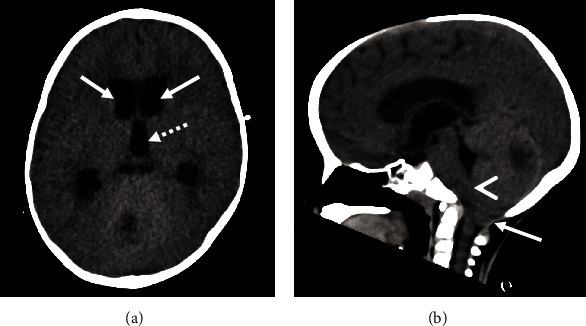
Noncontrast head CT images from hospital day 3 associated with neurological decline. (a) Axial CT image shows enlargement of the lateral (arrows) and third (dashed arrow) ventricles, and (b) midline sagittal CT image shows cerebellar swelling, effacement of the inferior fourth ventricle (arrowhead), and cerebellar tonsillar herniation (arrow).
